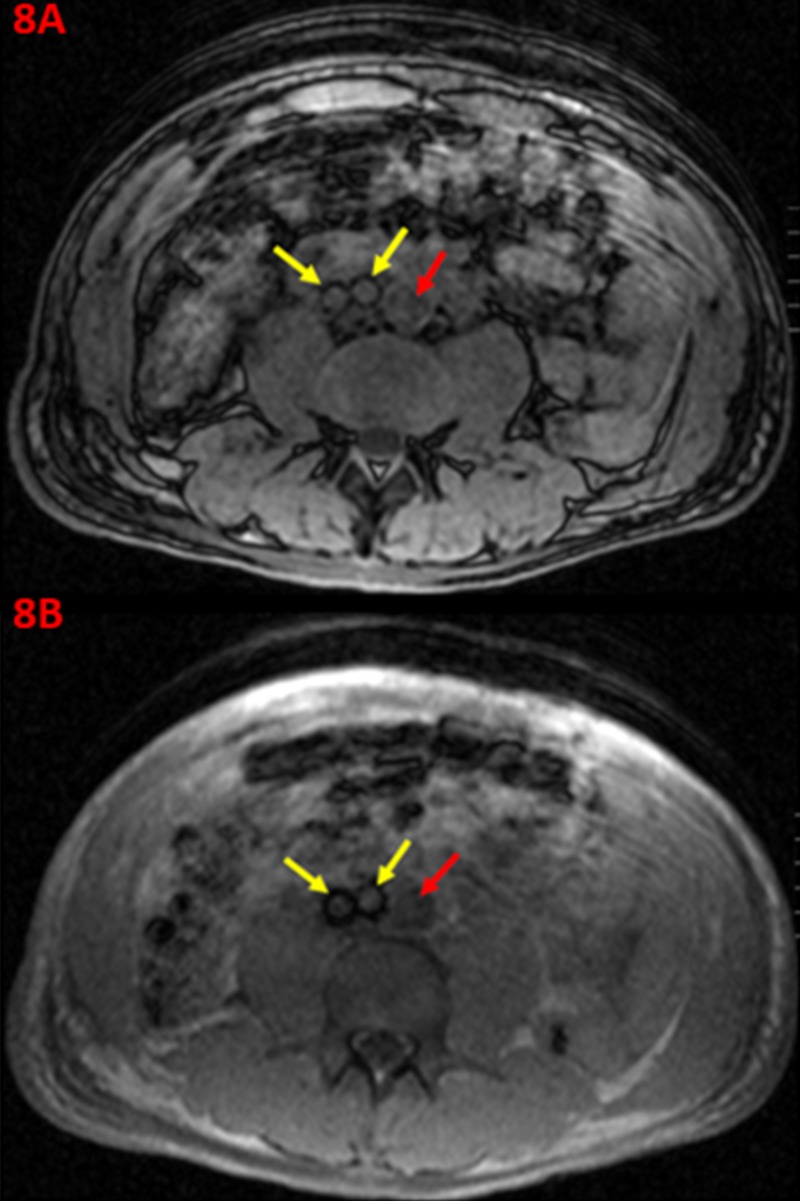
One-week Post-operative T1 Magnetic Resonance Images Displaying Stent Graft Patency within the Iliocaval Confluence A one-week post-operative axial T1 in/out of phase magnetic resonance image of the lower abdomen (8A) demonstrates patency of the right and left iliac veins (yellow arrows) and normal signal flow through the distal abdominal aorta (red arrow). An additional axial T1 in/out of phase magnetic resonance image of the lower abdomen at a slightly inferior axial cut (8B) demonstrates patency of the bilateral iliac veins (yellow arrows) with stent grafts visualized, and normal signal flow through the aorta (red arrow).
